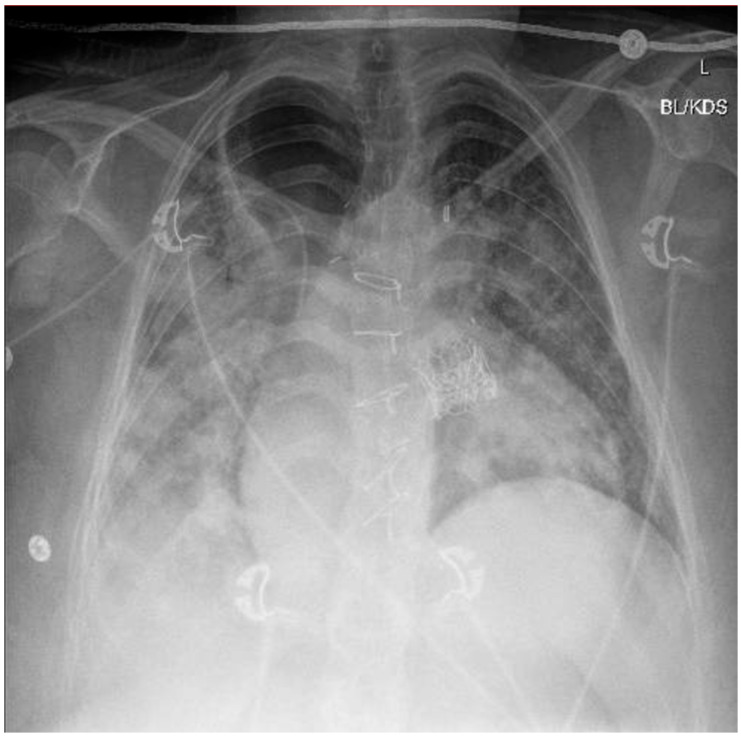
Postop chest X-ray (CXR) revealing increased multifocal airspace opacities most prominent in the right lung with a mediastinal shift and diffuse bilateral reticular opacities indicative of Lung Reperfusion Syndrome. Additionally median sternotomy wires from previous coronary artery bypass grafting (CABG) and TAVR can be noted.
